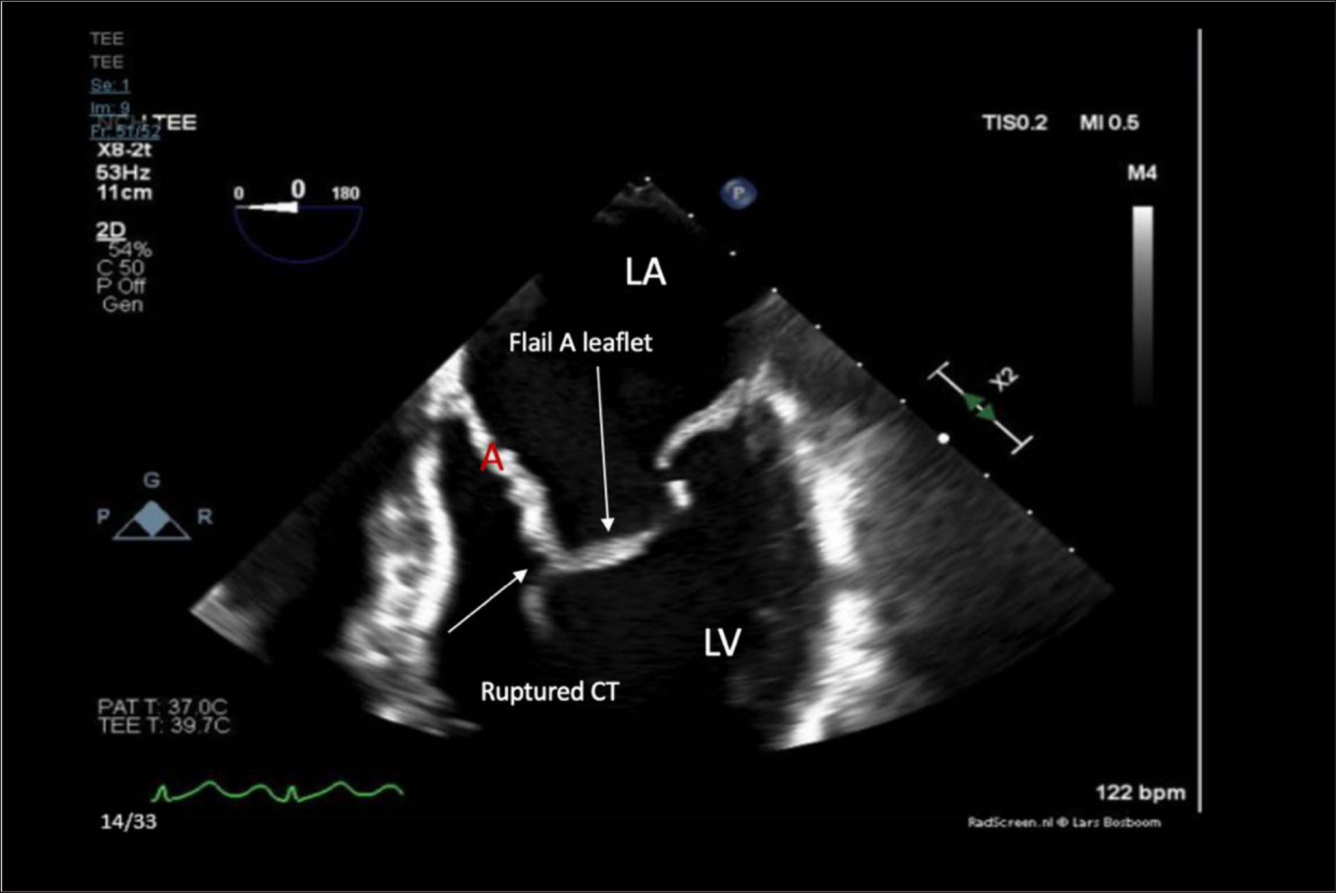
Zoomed in mid esophageal transesophageal echocardiogram with 4-apical view showing left ventricle (LV), left atrium (LA), chordae tendineae (CT), and flail (A) mitral valve leaflet.
